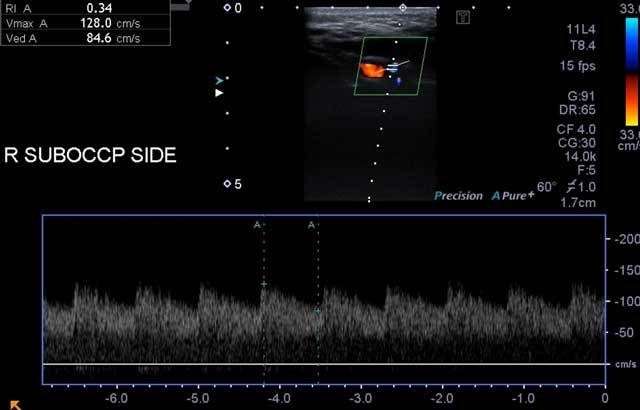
A vascular structure in the right suboccipital region showing high-speed, low-resistance blood flow pattern was confirmed with carotid Doppler sonography.
